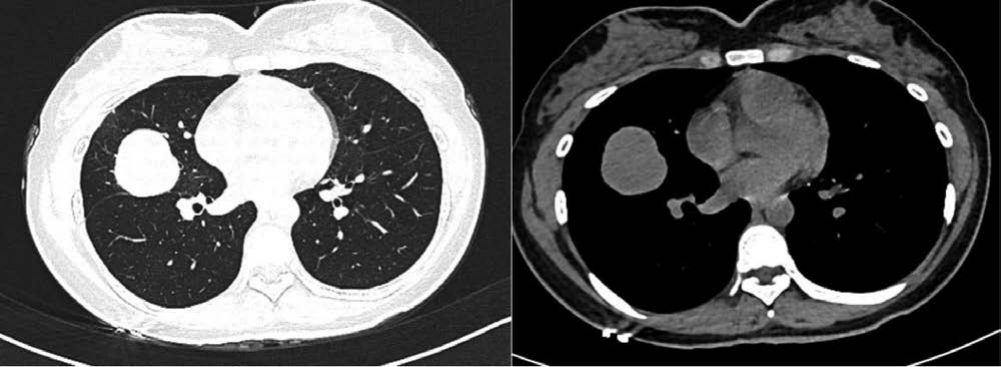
November 29, 2023 chest CT. CT = computed tomography.
